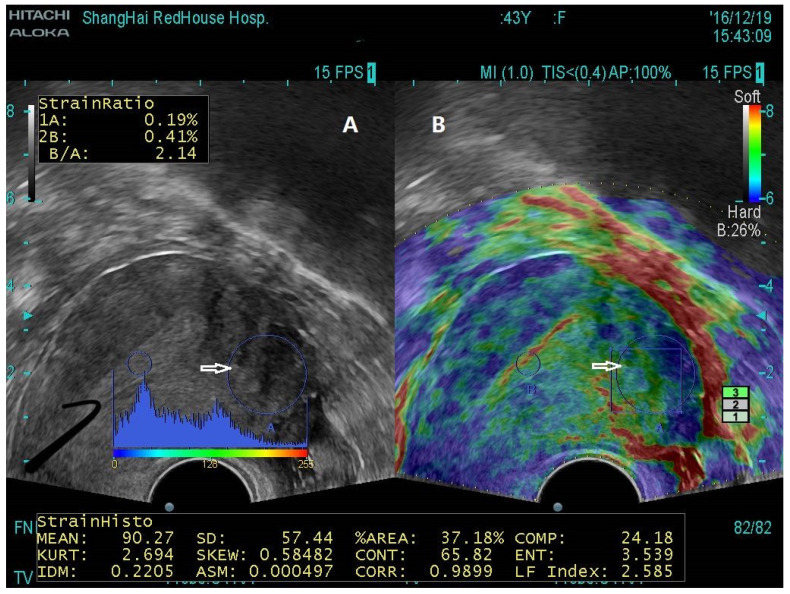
Transvaginal ultrasound image for a patient with a small uterus (59 × 58 × 57 mm) who complained of moderate dysmenorrhea with elevated CA125 level and was suspected with AM. (A) The big, circled area (white arrows) showed the ROI. The conventional B-mode TVUS image showed no sign that was consistent with a typical or spherical enlarged uterus or the presence of mild but not severe or obvious internal inhomogeneous echo in ROI. (B) Transvaginal elastosonography image showing an increased stiffness value (LFI = 2.585) in the same ROI shown in the TVUS (white arrow), indicative of adenomyosis. AM indicates adenomyosis; LFI, liver function index; ROI, region of interest; TVUS, transvaginal ultrasound. Replicated from Liu et al. [71] (Reprinted with permission from Reproductive Sciences).
